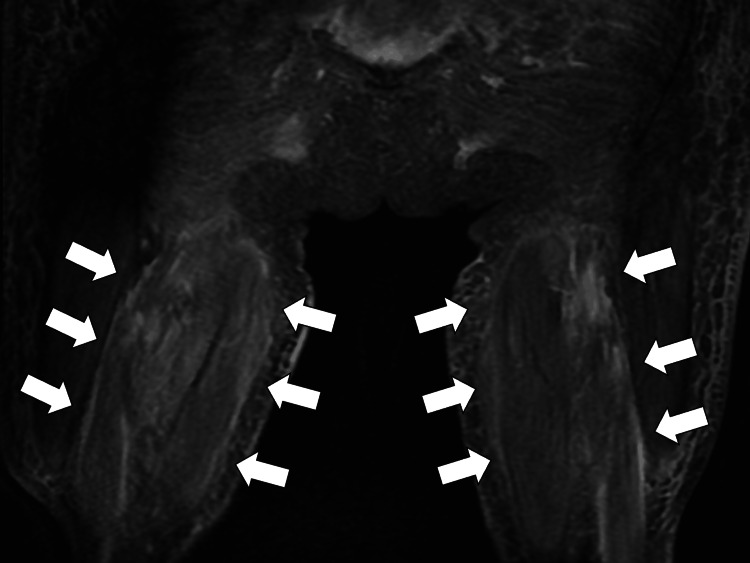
Lower limb muscle MRI (short tau inversion recovery) showing high signal intensity in bilateral quadriceps and biceps femoris (indicated by white arrows).
